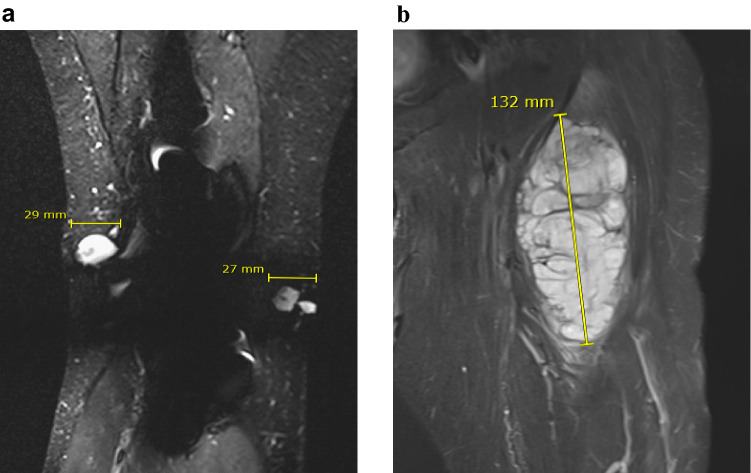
Magnetic resonance imaging shows recurrent tumor nodules around the knee prosthesis in (A), this tumor has been the source of *USZ20-EMC1.* A deep-seated multilocular tumor in the left thigh (B) high intensity on T2-weighted images has been the source for *USZ22-EMC2*
